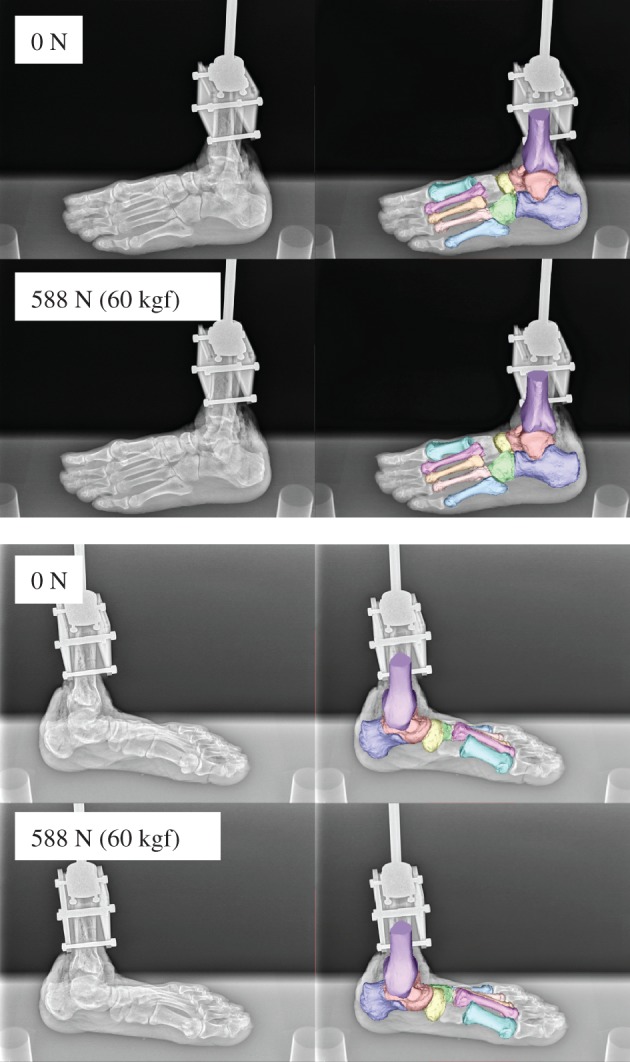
Biplane X-ray fluoroscopic images and corresponding registration results.
